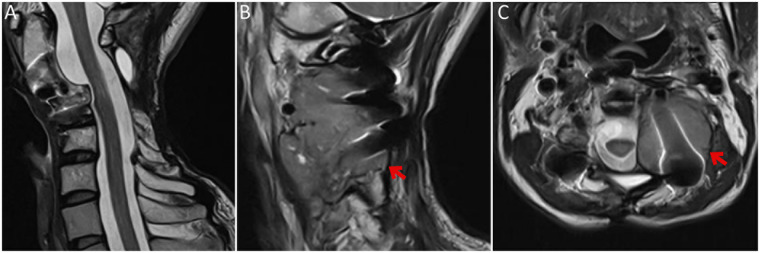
Preoperative cervical MRI in November 2025. T2-weighted images show (A) preserved cervical cord caliber and signal on a midline sagittal view, (B) an off-midline sagittal view demonstrating a recurrent left cervical paraspinal mass, and (C) an axial T2-weighted image showing dural indentation despite susceptibility artifact from prior instrumentation. Red arrows indicate the tumor in (B,C).
